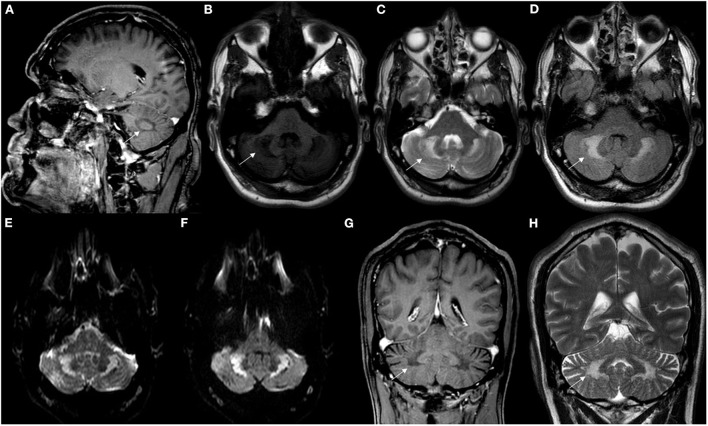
Neuroimaging patterns observed in CTX. (A) Sagittal T1-weighted brain MRI showed hypointense signal in deep cerebellar white matter (white arrow). Axial brain MRI disclosed signal change in deep cerebellar white matter (white arrows) hypointense in T1W (B) and hyperintense in T2W (C) and FLAIR sequences (D). Axial brain MRI disclosed hyperintensity in deep cerebellar white matter in DWI (E) and ADC sequences (F). Coronal brain MRI showed hypointensity in the deep white matter in T1W imaging (G), as well as corresponding hyperintensity (white arrows) in T2W imaging (H).
